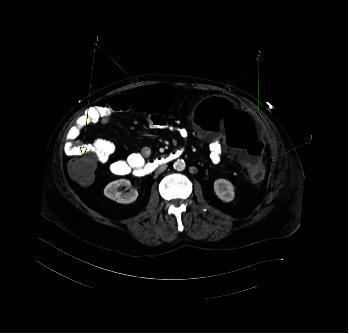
CT scan with normal proximal colon wall thickness (1). Distal transverse colon and proximal descending colon wall thickening and hypoenhancement suspicious for ischemic colitis (2). Point of partial colon obstruction and no visible obstructive colon, omental, or mesenteric mass (3). CT, computed tomography.
